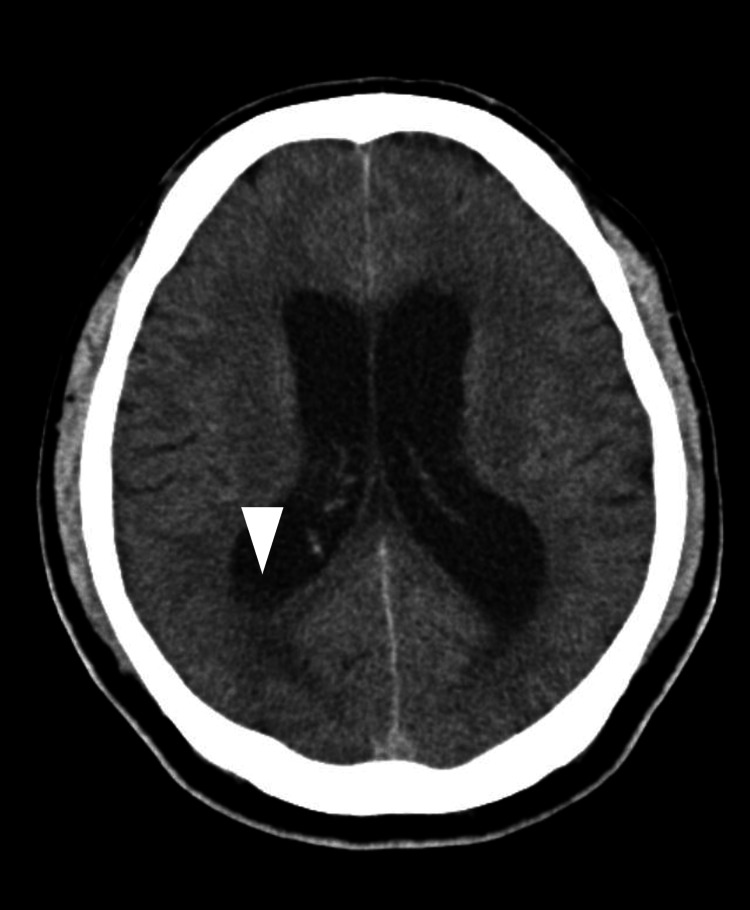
Brain CT at admission. The initial CT scan demonstrated enlargement of the bilateral lateral ventricles with subtle fluid accumulation in the right lateral ventricle (white arrowhead).
